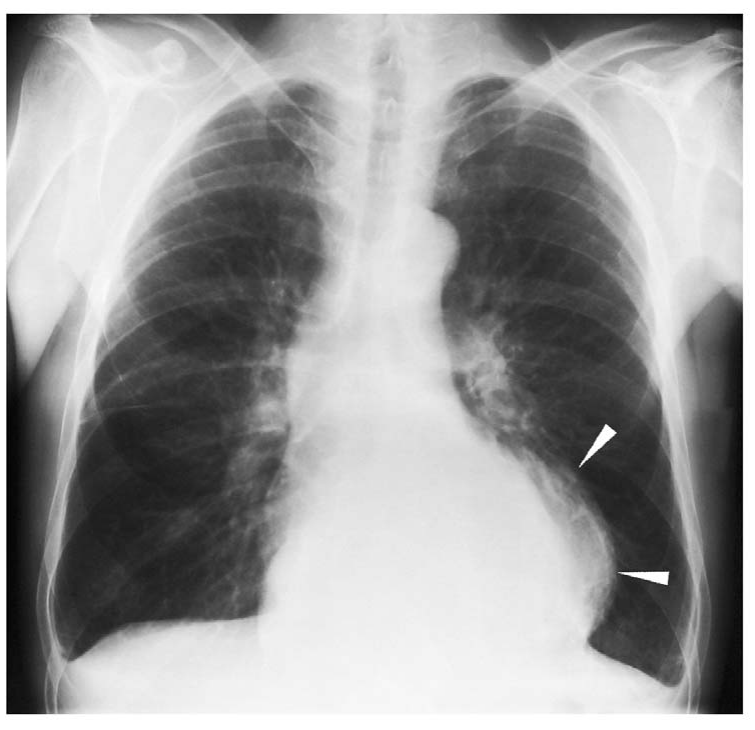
Chest X-ray on admission. Initial examination showed an intrathoracic mass overlying the left margin of the heart (arrowheads). No interstitial pulmonary edema was noted. Small pleural effusions are shown.
